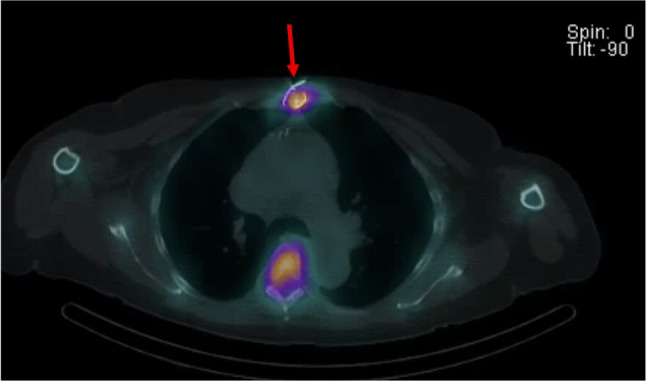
Axial SPECT CT image demonstrating high signal within the sternum
